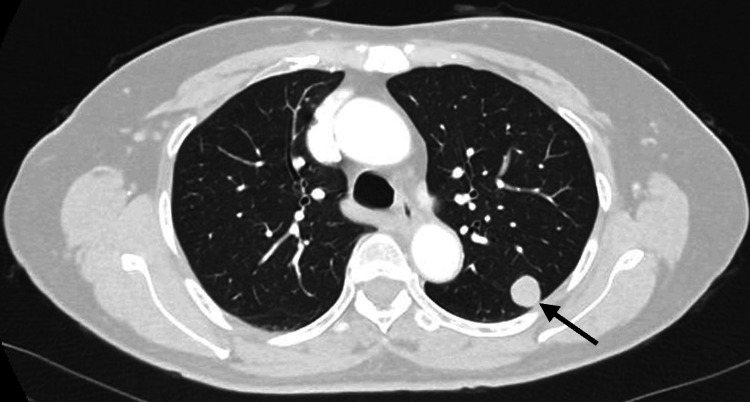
CT scan showing the 16 mm nodule (arrow) in the left lung
